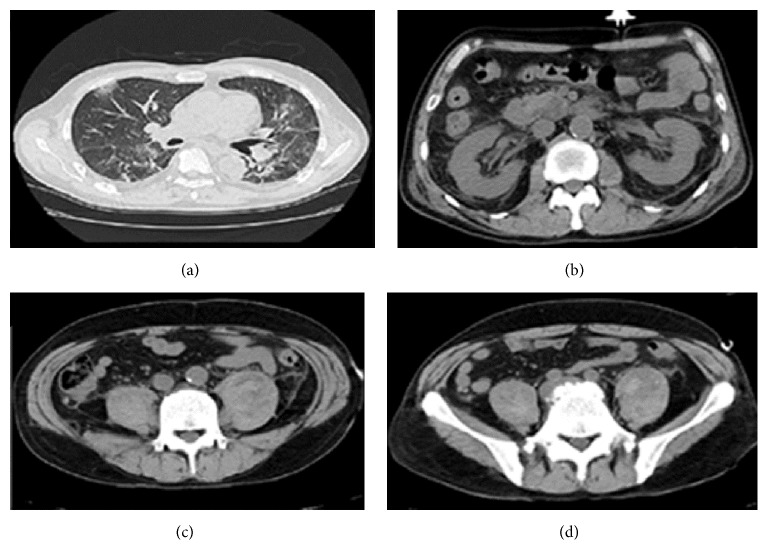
Chest and abdominal computed tomography findings. (a) Mild pneumonia and alveolar hemorrhage. (b) Edema in the retroperitoneum surrounding the kidney. (c) and (d) Bilateral enlargement of the iliopsoas muscles and areas of nonuniform swelling.
